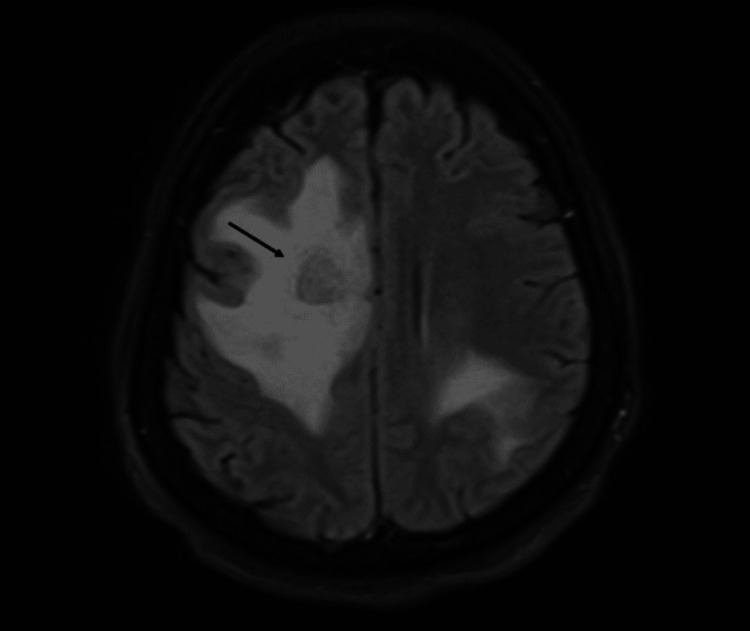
MRI from September 2022 showing intracranial metastases with intra-axial cortico-subcortical localization and supratentorial distribution in both cerebral hemispheres. Extensive vasogenic edema is present, predominantly affecting the right frontal lobe
